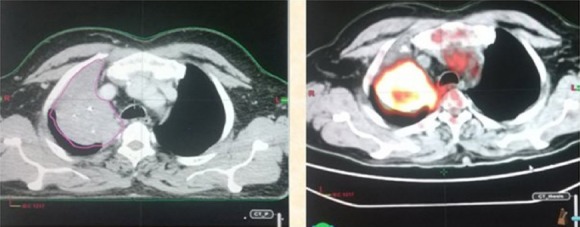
GTV primary tumor volume drawn in magenta on CECT and red on ^18^F-FDG PET-CT showing decrease in GTV volume on ^18^F-FDG PET-CT
